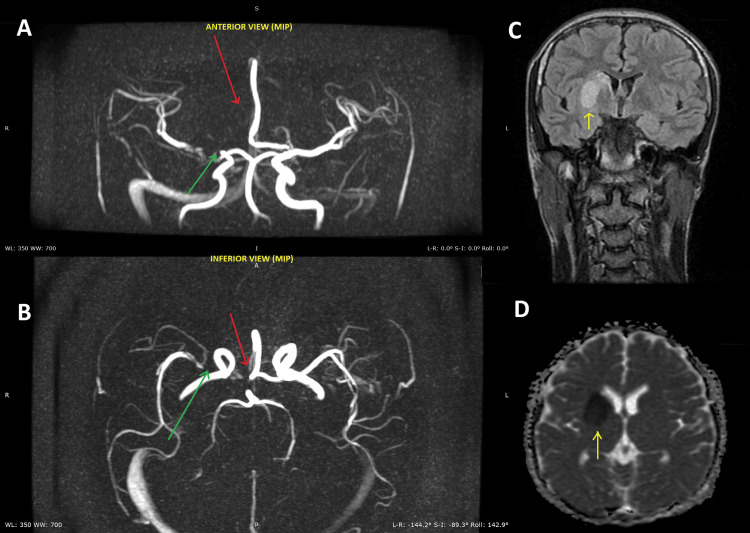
Brain MRI and MRA demonstrating acute basal ganglia infarction with congenital agenesis of the right distal internal carotid artery and right anterior cerebral artery (A, B) Maximum intensity projection (MIP) MRA images in anterior (A) and inferior (B) views demonstrating absent visualization of the right distal internal carotid artery (green arrow) and right anterior cerebral artery (red arrow). (C) Coronal T2-weighted MRI showing a heterogeneously hyperintense lesion in the basal ganglia (yellow arrow). (D) Axial T1-weighted MRI showing a corresponding focal hypointense lesion in the basal ganglia (yellow arrow).
